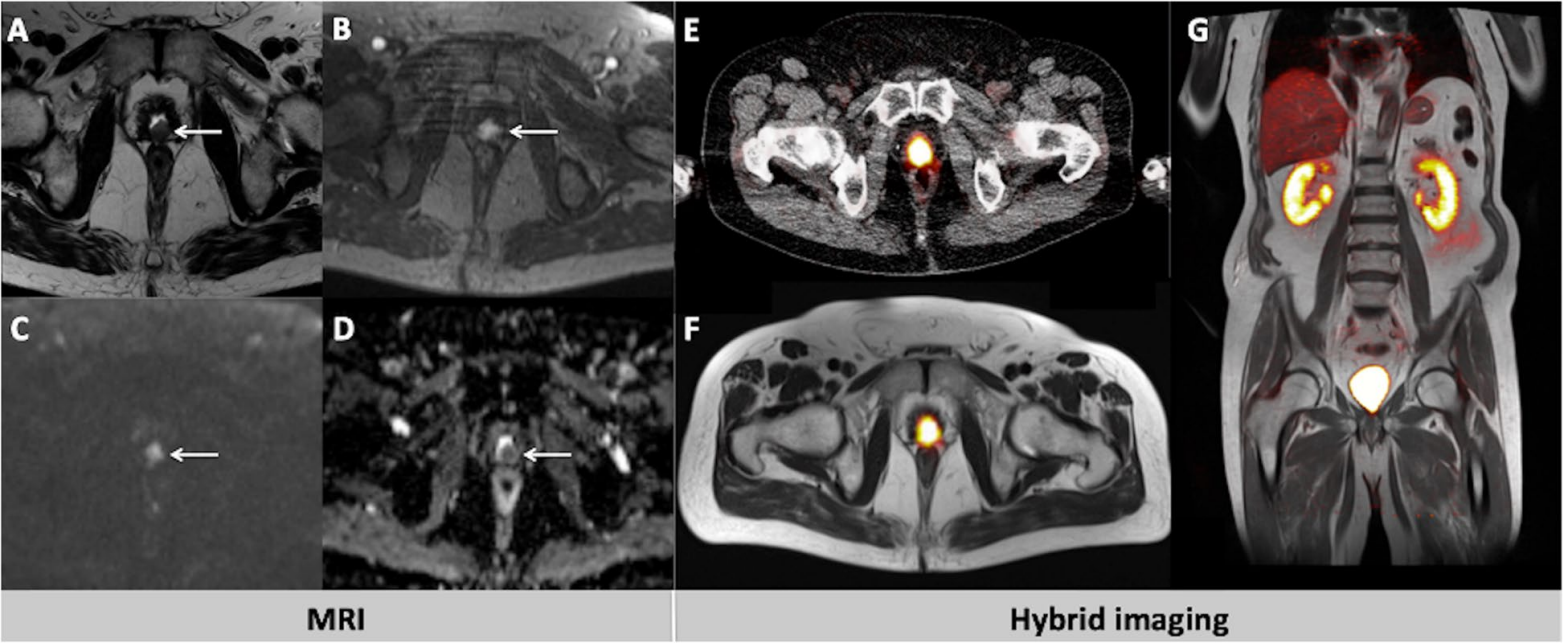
A 77-year old patient with biochemical recurrence of PCa after radical prostatectomy. His PSA level at the time of examination was 2.0 ng/ml. In MRI, a lesion in the prostate bed with hypointense T2w signal, suspicious for PCa local recurrence is depicted (A, arrow). The lesion shows marked early contrast enhancement in the dynamic T1w (B, arrow) and distinct diffusion restriction with signal increase in the b1500 image (C, arrow) and corresponding signal decrease in ADC map (D, arrow). In the hybrid imaging modalities PET-CT (E) and PET-MRI (F, G), the suspicious lesion is overseen due to its masking by intensive tracer accumulation in the urinary bladder
